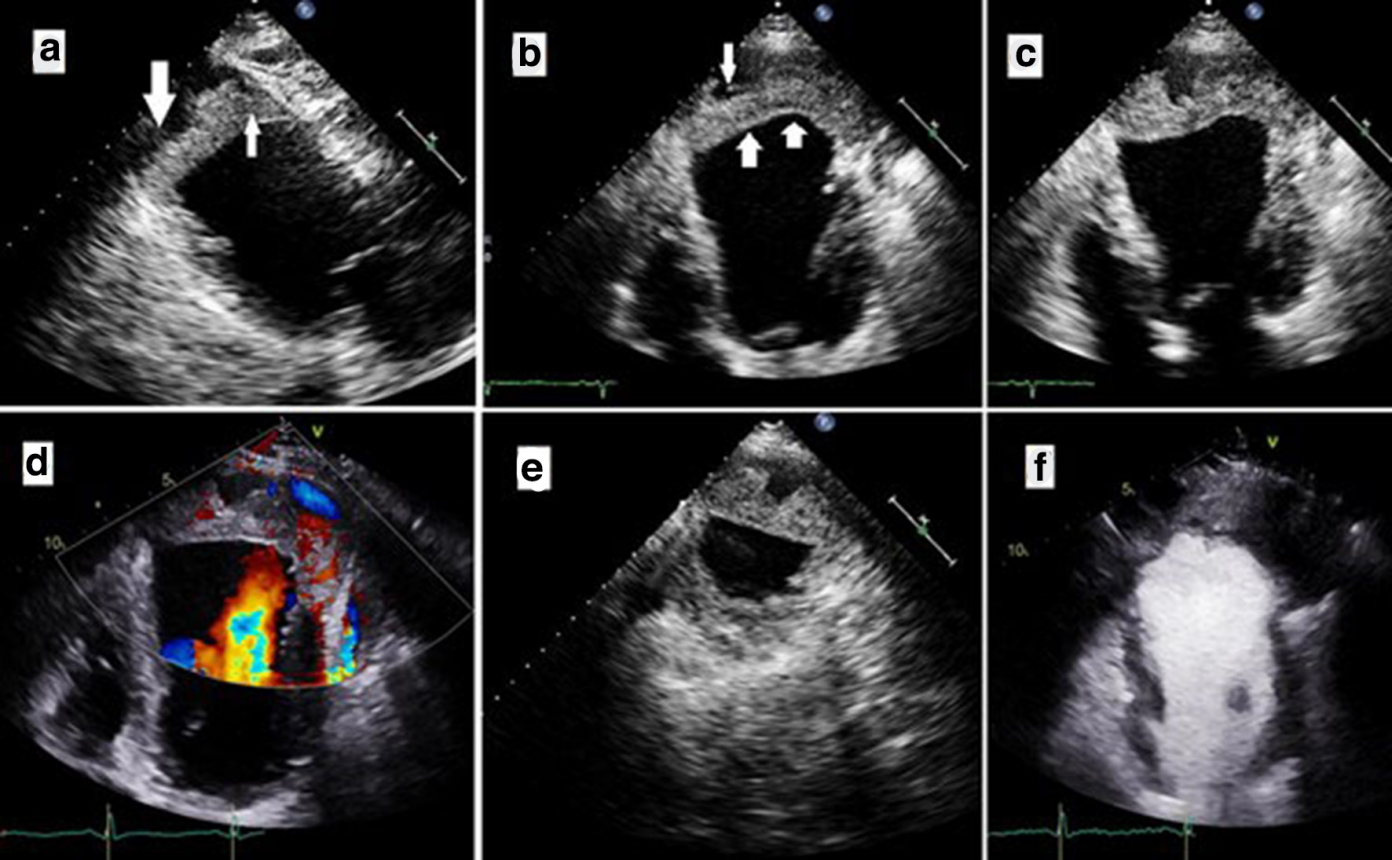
Transthoracic echocardiography: Parasternal long-axis view (a). Apical four-chamber view in systole (b) & diastole (c).Colour Doppler echo (d). Apical parasternal short-axis view (e). Contrast echo (f). LV apical partially thrombosedneocavity (arrows in a, b), the inner boundary of which is made by an endomyocardial layer displaying paradoxical diastolic contraction (c).No detectable communication between the LV cavity and the neocavity neither in the colour Doppler nor the contrast echo images. LV ( left ventricle).
